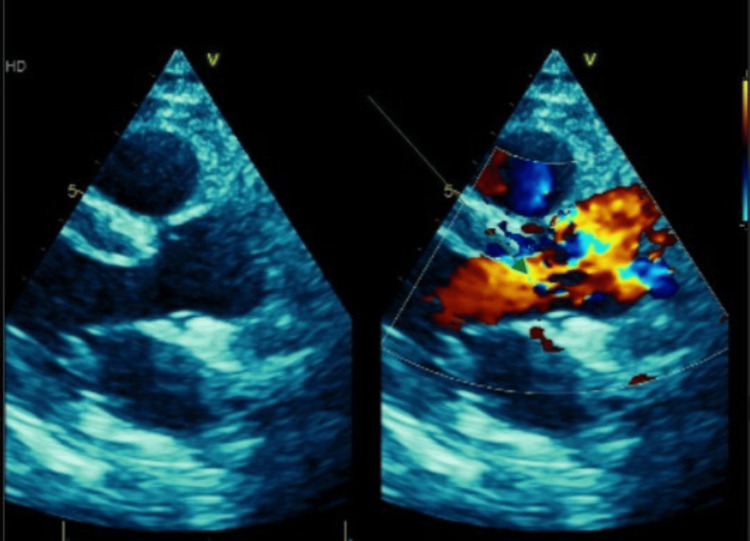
Transthoracic echocardiography showed normal systolic function, no intracardiac thrombus, and no patent foramen oval. Mild aortic regurgitation was observed (green arrow).
